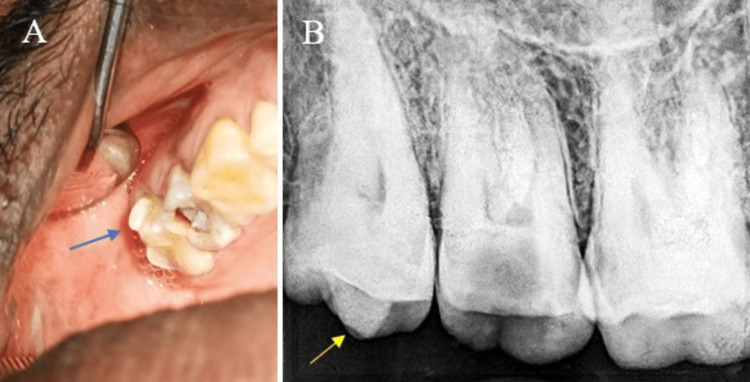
A. Intraoral examination revealed a Talon-cusp on the buccal surface of the Maxillary second molar. B. Intraoral periapical radiograph revealed a Talon-cusp-like structure in the crown of the Maxillary second molar
